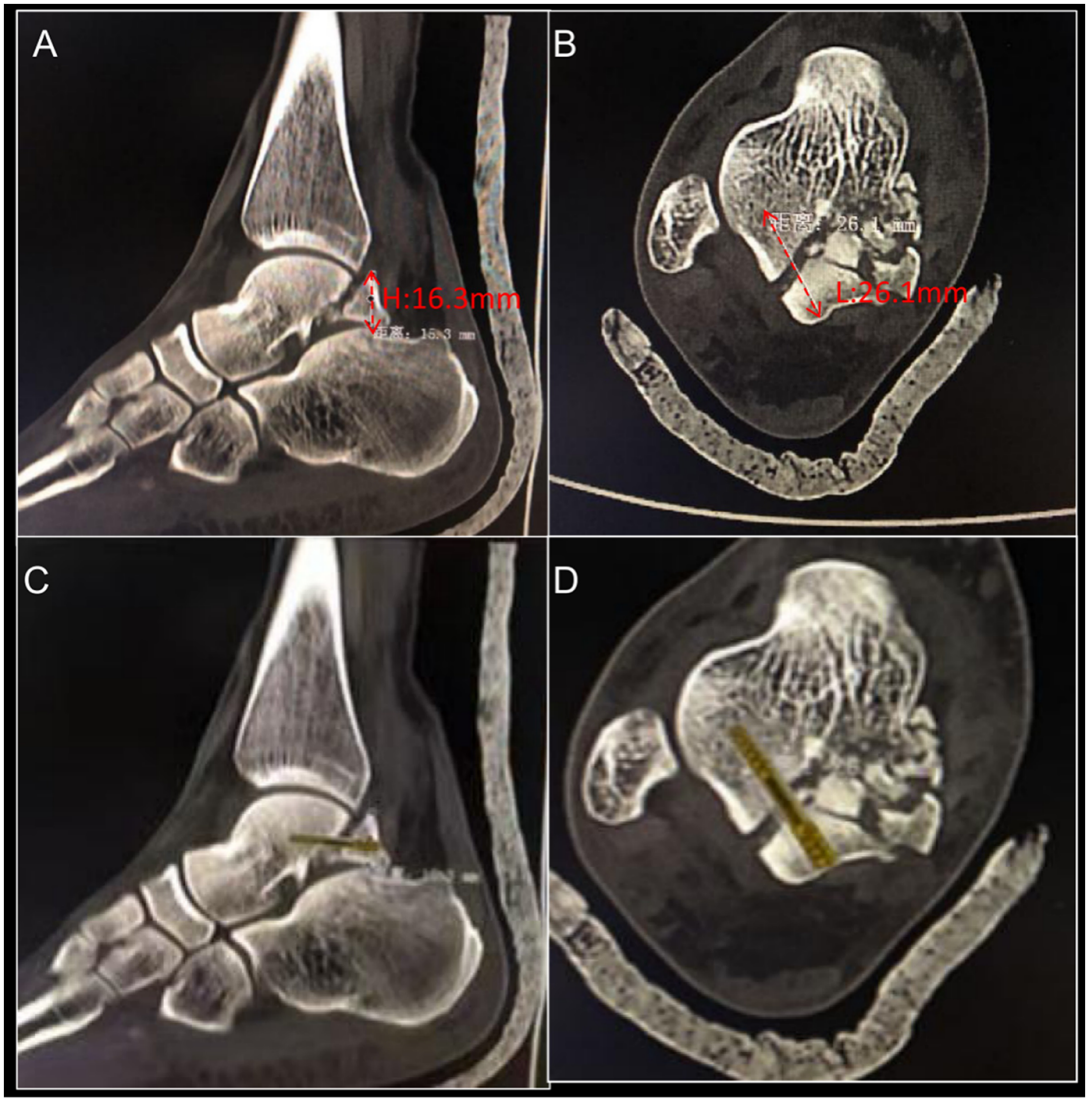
(A) CT sagittal view showing the fracture fragment with a height of 16.3 mm. (B) CT axial view showing the screw fixation length of 26.1 mm. CT sagittal view (C) and axial view (D) with fitted Herbert screw, determining the screw length and surgical plan.
